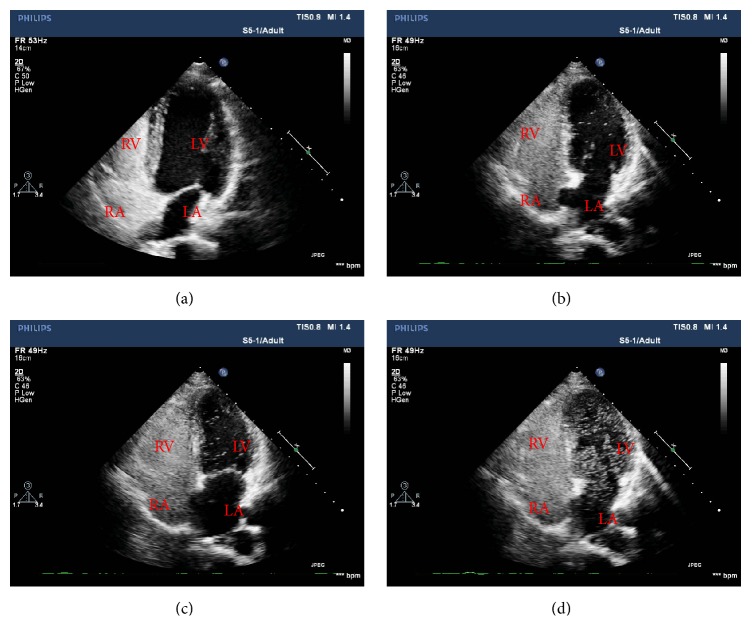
Semiquantitative grading of a patent foramen ovale-right-to-left shunt by transthoracic echocardiography using the bubble test. (a) No right-to-left shunt. (b) Mild right-to-left shunt (1–10 microbubbles in the left atrium). (c) Moderate right-to-left shunt (11–30 microbubbles in the left atrium). (d) Extensive right-to-left shunt (more than 30 microbubbles in the left atrium or left atrial opacity). LA = left atrium; RA = right atrium; LV = left ventricle; RV = right ventricle.
